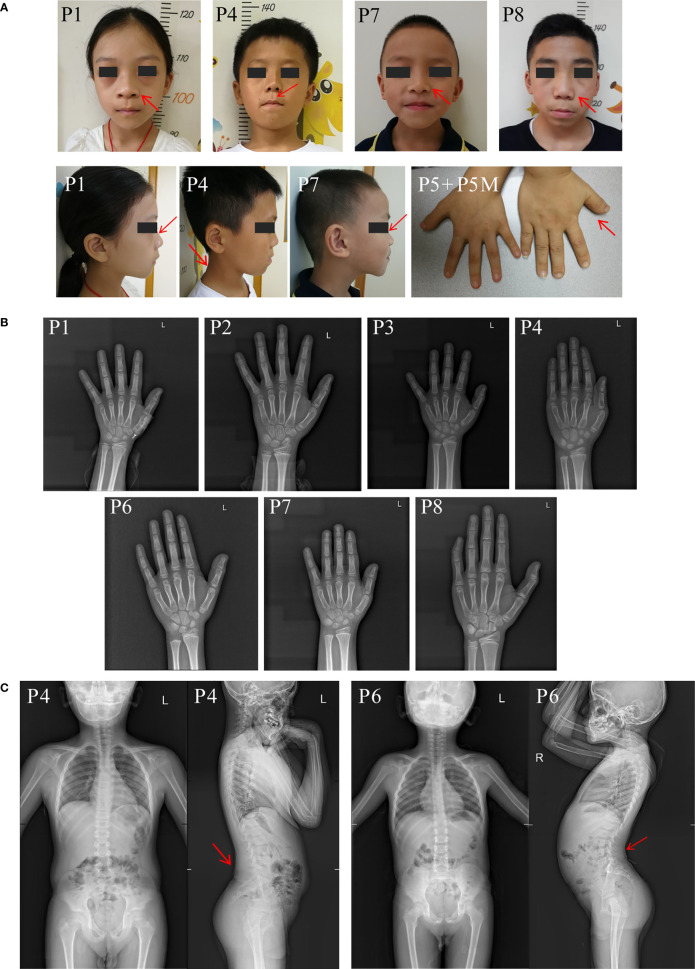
Clinical characteristics of the probands in our center. (A) Clinical pictures of our patients and their affected families. Red arrow symbols indicated the mild dysmorphic features of the patients. Patient numbers were indicated on the left upper corner of each plot. (B) Bone age radiographs of some affected patients’ left hands. (C) Spinal radiographs of probands patient 4 and patient 6. The patient’s identification is indicated in the top-left corner.
